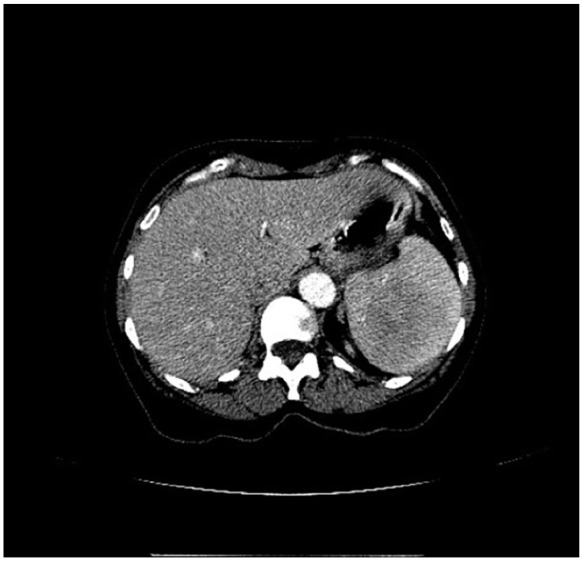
Transaxial* view of abdominal CT. Indication of enlarging splenic mass within the superior midportion of the spleen.
